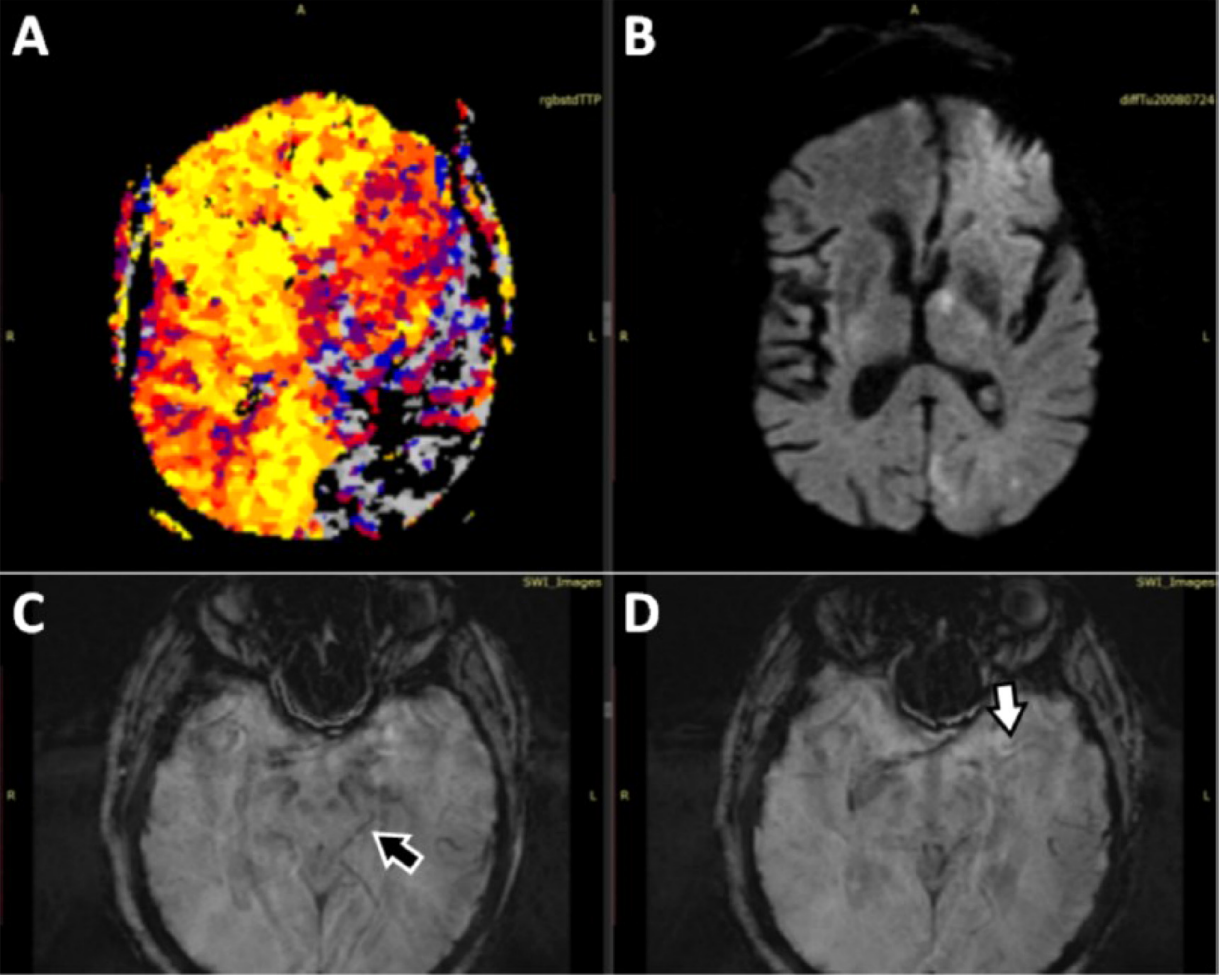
Case 2 - Geometrically matched stdTTP- (A*: regions with a critical residual perfusion appear grey, while black colored regions mark areas with a perfusion stop*) and DWI-maps (B) of case 2 showed a significant mismatch in the left occipital and temporal lobe, rather suggesting acute ischemia in the left PCA-territory. No thrombus material was detectable in SWI, neither in the course of the fPCA (C*: black arrow*) nor the MCA on the left side (D*: white arrow*). DWI, diffusion weighted; fPCA, fetal posterior cerebral artery; MCA, middle cerebral artery; stdTTP, standardized time-to-peak parameter; SWI, susceptibility weighted.
